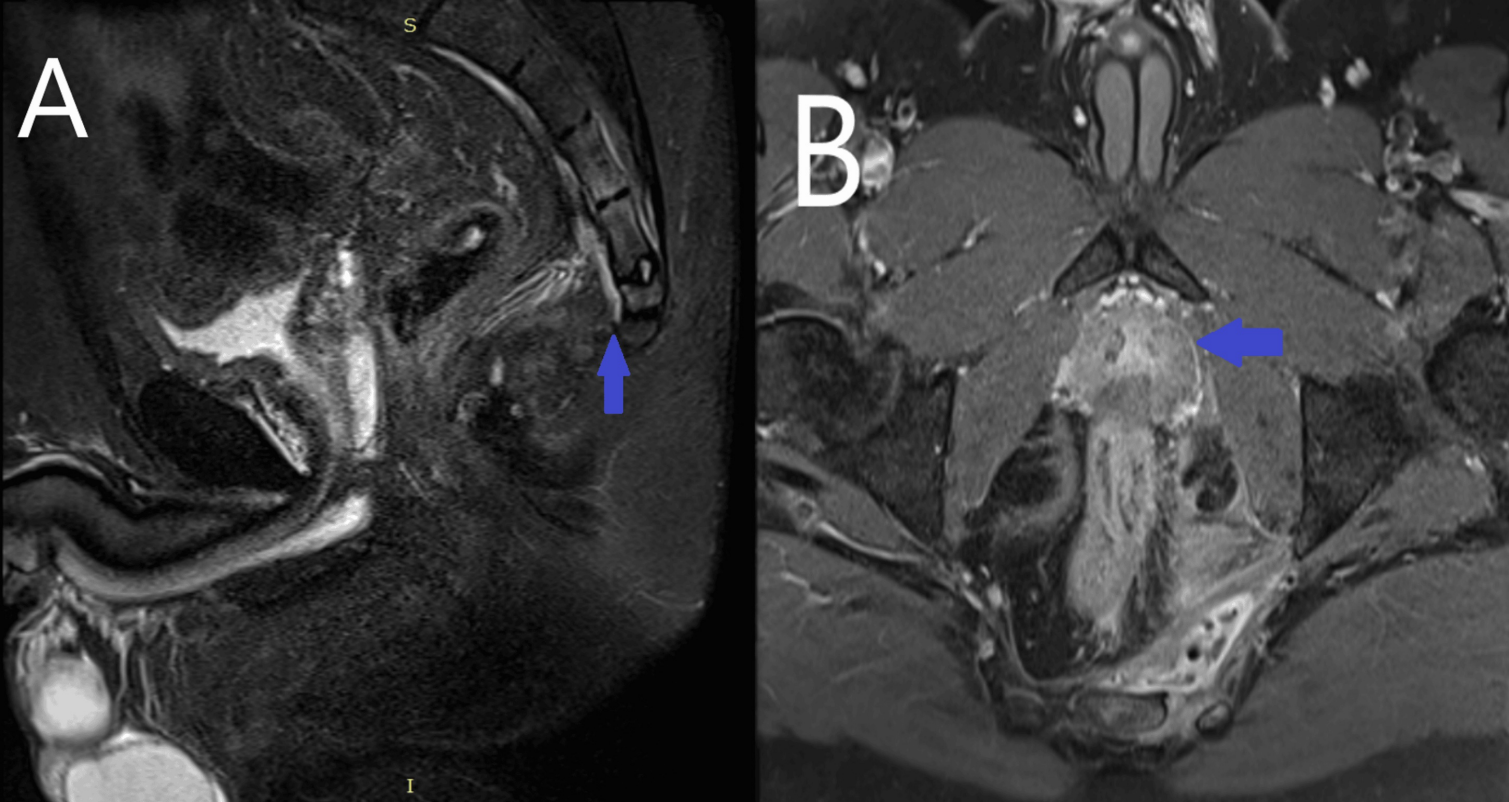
Preoperative MRI images A: On the sagittal short tau inversion recovery (STIR) image, intramedullary hyperintensity consistent with osteomyelitis was observed on the opposite surfaces of the sacral 4-5 vertebrae. Inflammatory hyperintense soft tissue thickening was also noted in the presacral fat tissue. B: On the post-contrast T1 fat-saturated transverse image, an abscess formation containing air with an irregular enhancing wall was seen in the midline-left side of the presacral area, and enhancement consistent with osteomyelitis was noted in the adjacent bone.
